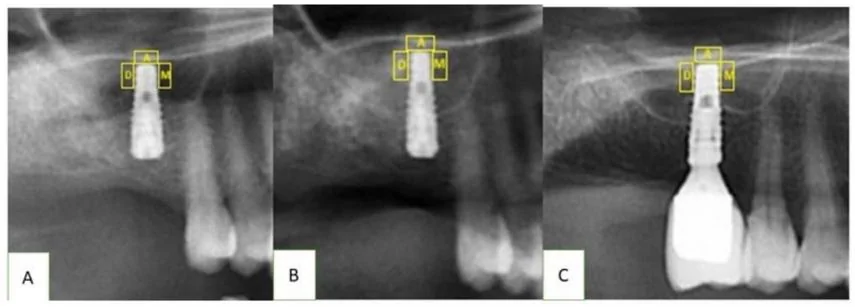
Representative placement of the regions of interest on cropped panoramic radiographs. (A–C) show the baseline, postoperative 6-month, and postoperative 12-month radiographs, respectively. The ROI labels A, M, and D denote the apical, mesial, and distal regions, respectively. The implant long axis, apex, and mesial and distal surfaces were used as fixed reference landmarks to reproduce ROI placement across the three time points. ROI dimensions and orientations were maintained unchanged.
